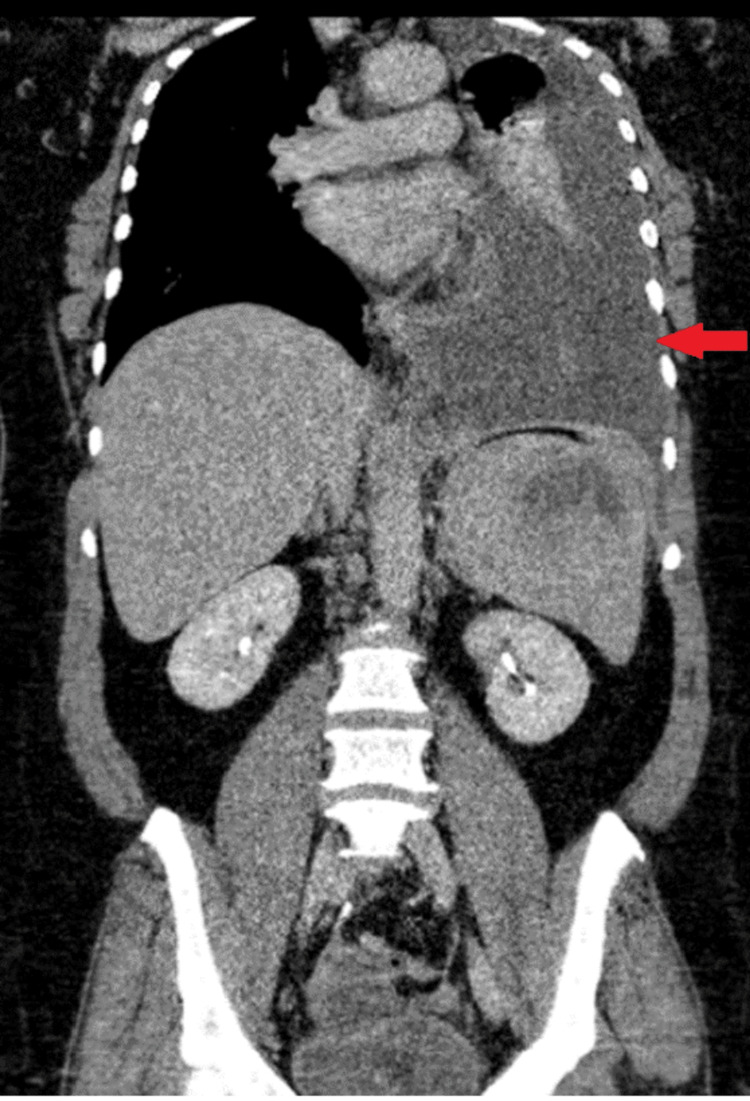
Follow-up CT abdomen with IV contrast in the coronal view after three months showed rapid development of large left-sided pleural effusion (red arrow) with passive collapse consolidation of left lung lobes, with no signs of mediastinal lymph nodes.
